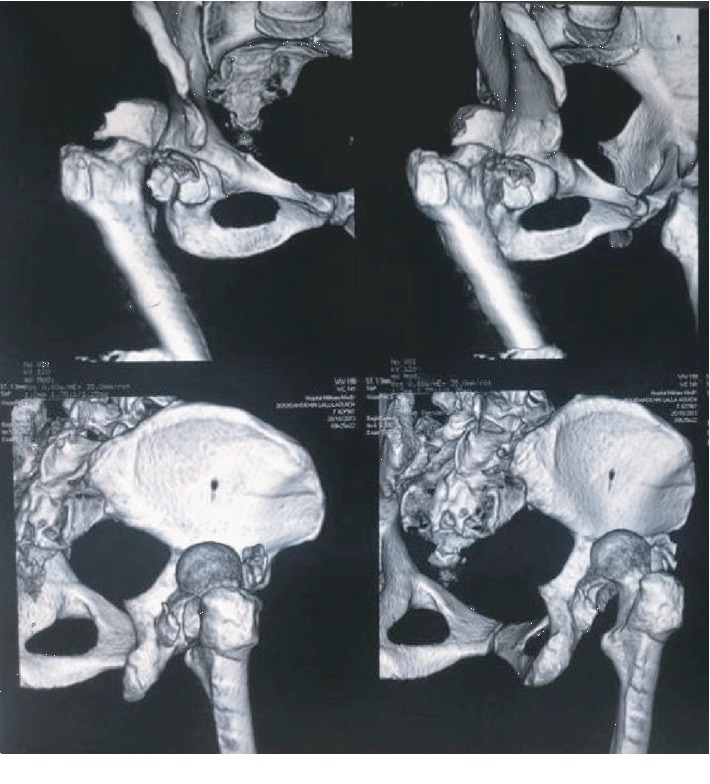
Three-dimensional computed tomography images showing a dislocation fracture of the right acetabulum.
